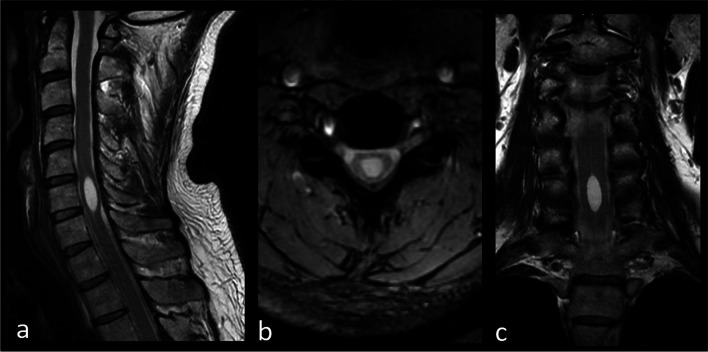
Cervical spine MRI study. T2-weighted images on sagittal (a), axial (b) and coronal (c) planes. The figure shows a focal fluid-filled cavity centrally located within the spinal cord at C6 level. This dilation is lined by the normal ependymal lining of the central canal and corresponds to a hydromyelic cavity
